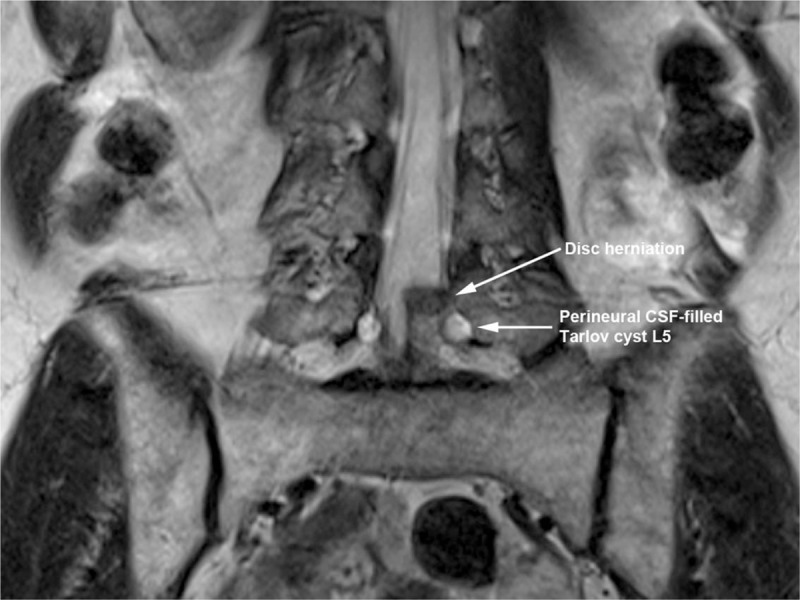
Left-sided disc herniation at L4–5 and foraminal Tarlov cysts in close proximity to approximate needle trajectory for transforaminal epidural injection technique on coronal T2-weighted magnetic resonance imaging.
